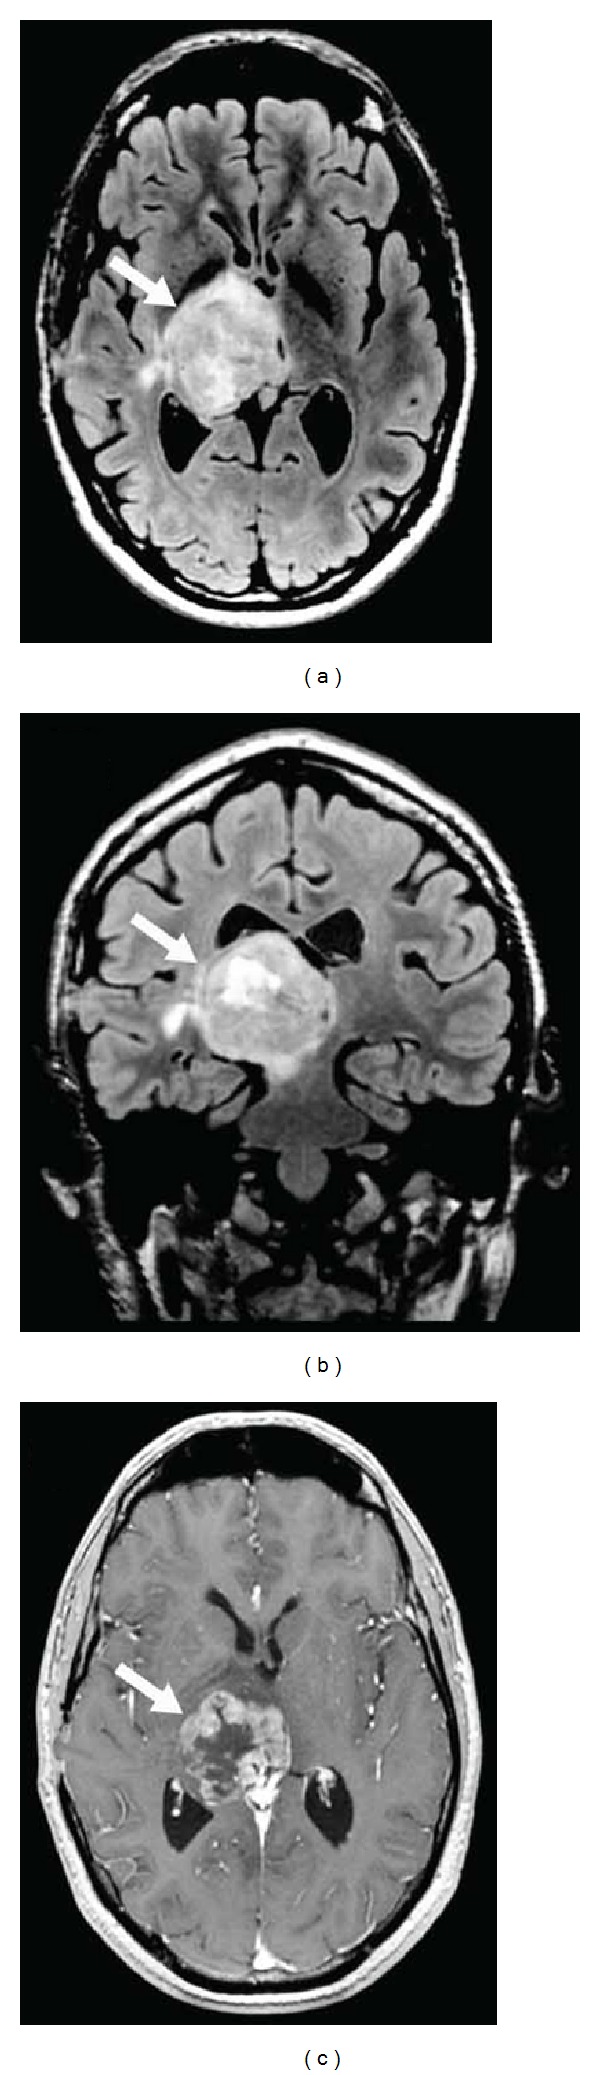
A patient with glioblastoma multiforme in the right thalamus seen as hyperintensity on axial (a) and coronal (b) FLAIR imaging, with gadolinium-enhancement on axial T1-weighted imaging (c).
